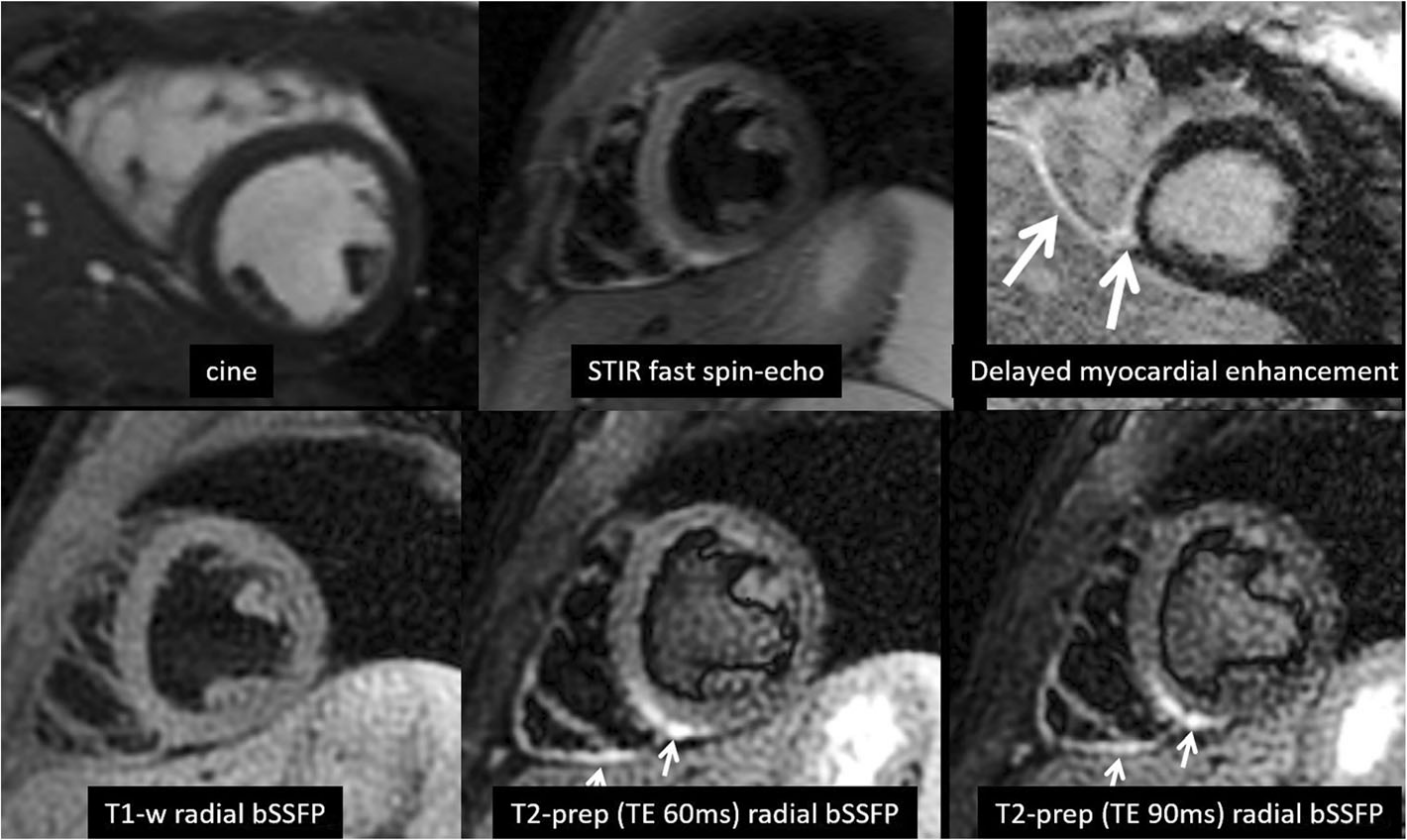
Patient presenting with chest pain and CMR findings consistent with myocarditis. Top row (from *left* to *right*): cine, T2-weighted STIR fast spin-echo, myocardial delayed enhancement using inversion recovery spoiled gradient-echo. Bottom row (from *left* to *right*): T1-weighted radial bSSFP, T2-weighted radial bSSFP with T2_prep_ time = 60 ms, T2-weighted radial bSSFP with T2_prep_ time = 90 ms. The T2-signal abnormality involving the inferior walls of the left and right ventricles is seen in the T2-weighted STIR fast spin-echo image, but is better delineated with T2-weighted radial bSSFP. Right ventricular trabeculations are also better delineated with the radial acquisitions. In this example, the blood pool was effectively nulled in the T2-weighted STIR fast spin-echo and T1-weighted radial bSSFP images, whereas the left ventricular blood pool has intermediate signal intensity in the T2-weighted radial bSSFP image due to suboptimal setting of the time delay following the dual-inversion preparation
